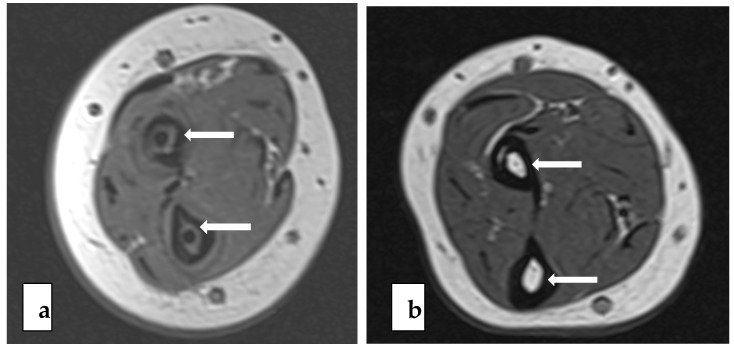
T1-weighted axial images showing low-signal biodegradable nails (white arrows) one month postoperatively (a) and faint nail residuals 6.5 years postoperatively (b).
